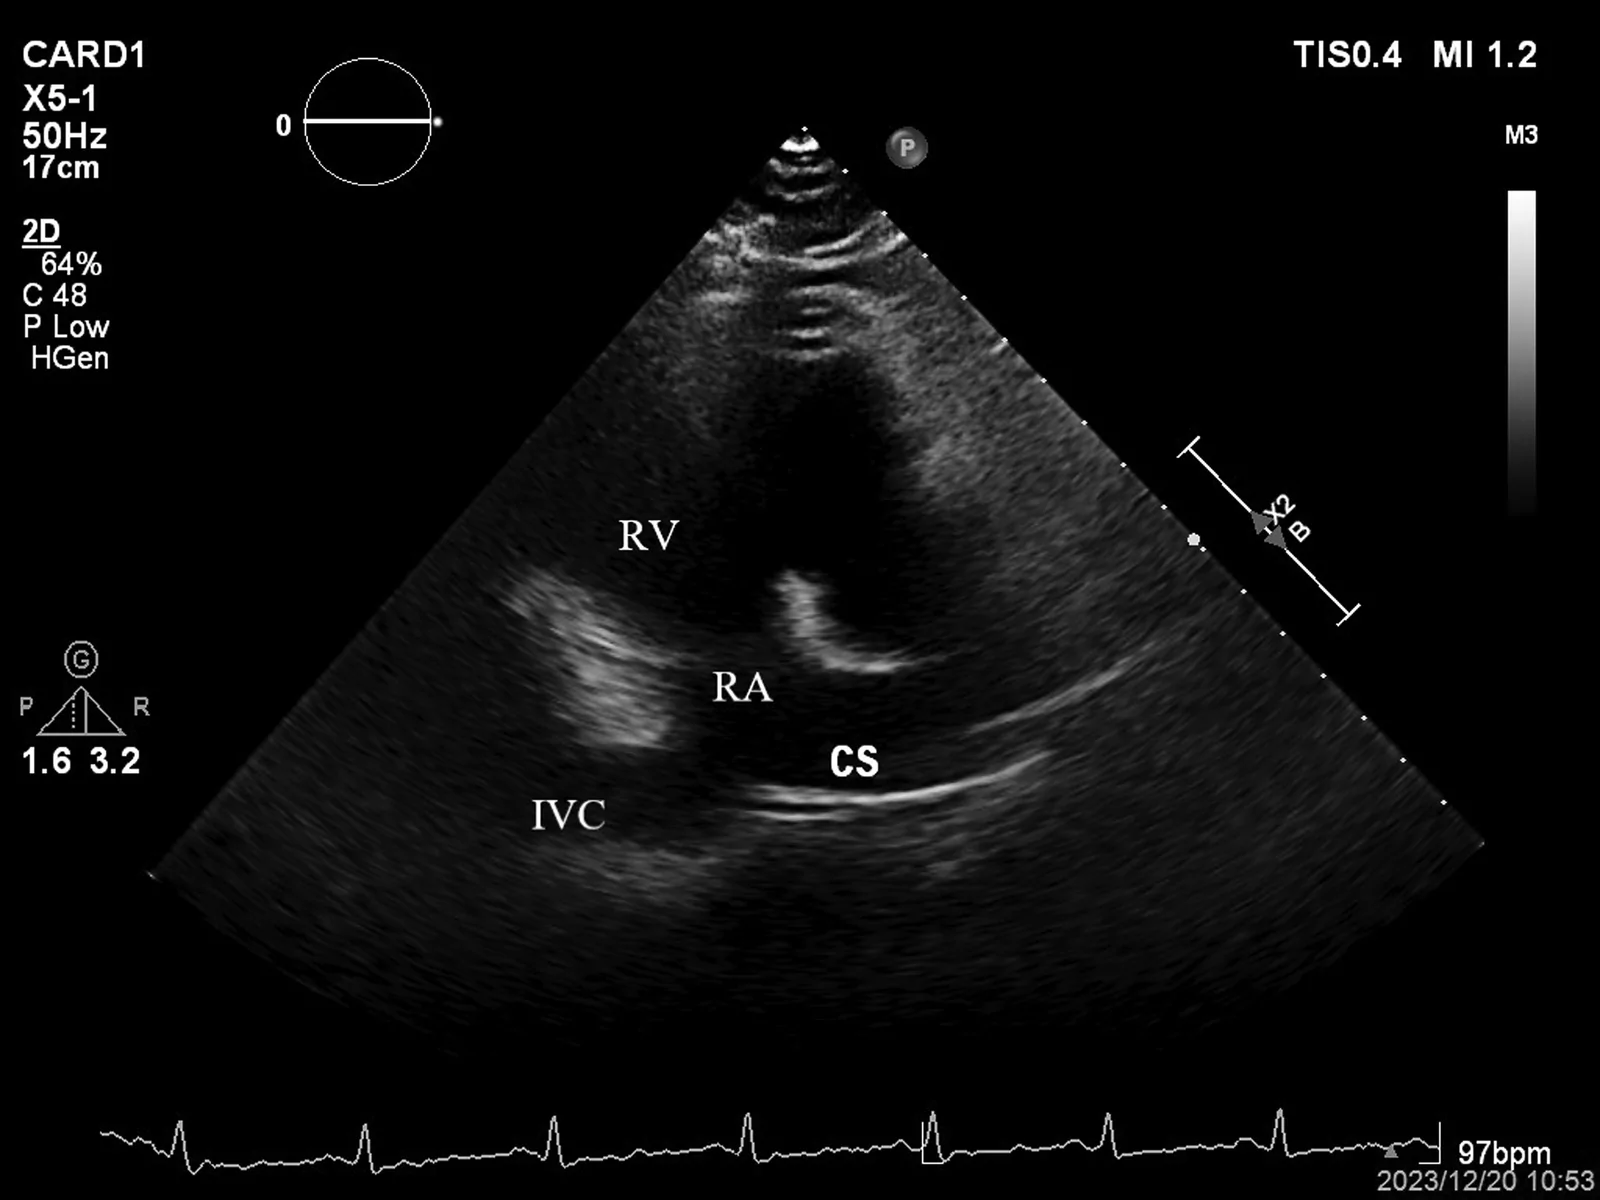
Two-dimensional transthoracic echocardiogram, apical view, showing coronary sinus enlargement (anteroposterior diameter 21 mm). CS = coronary sinus, IVC = inferior vena cava, RA = right atrium, RV = right ventricle.
